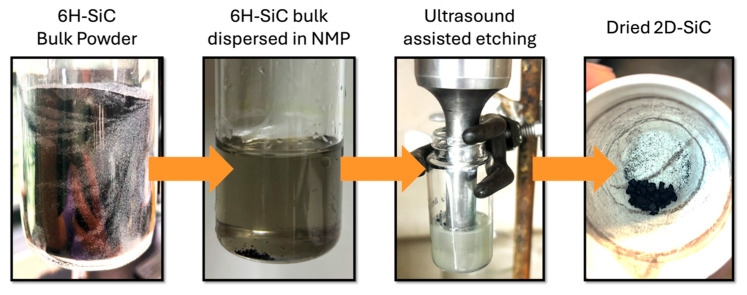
Step-by-step photographs of the ultrasonic-assisted wet exfoliation of bulk 6H–SiC powder using the Cole-Palmer vertical probe sonicator.
